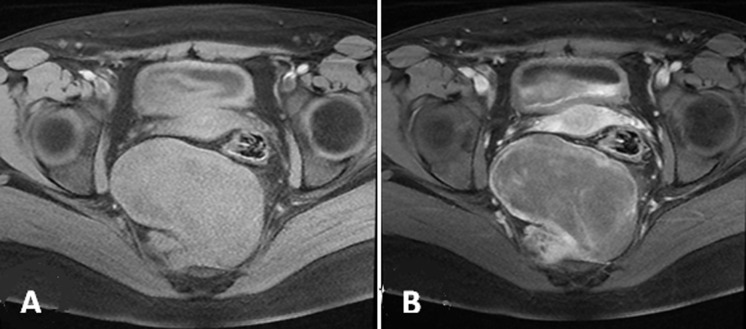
pelvic MRI T1-weighted images FAT-SAT: A) pre gadolinium; B) post gadolinium
